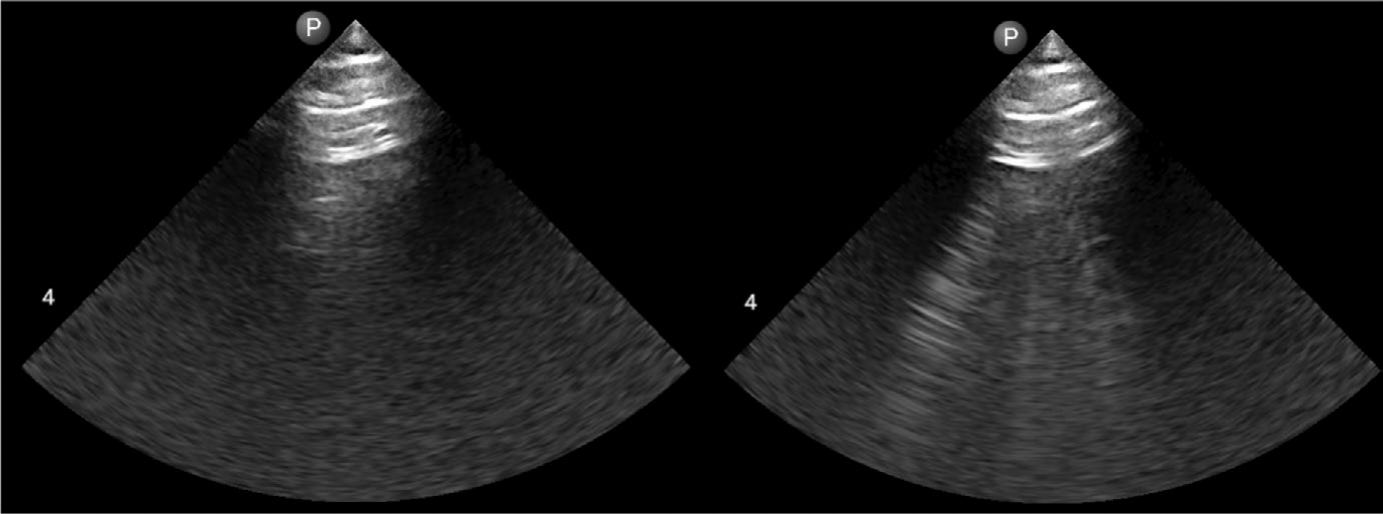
Lung ultrasound in a single participant on visit 1, pre (left) and post (right) exercise that developed mild pulmonary edema.
